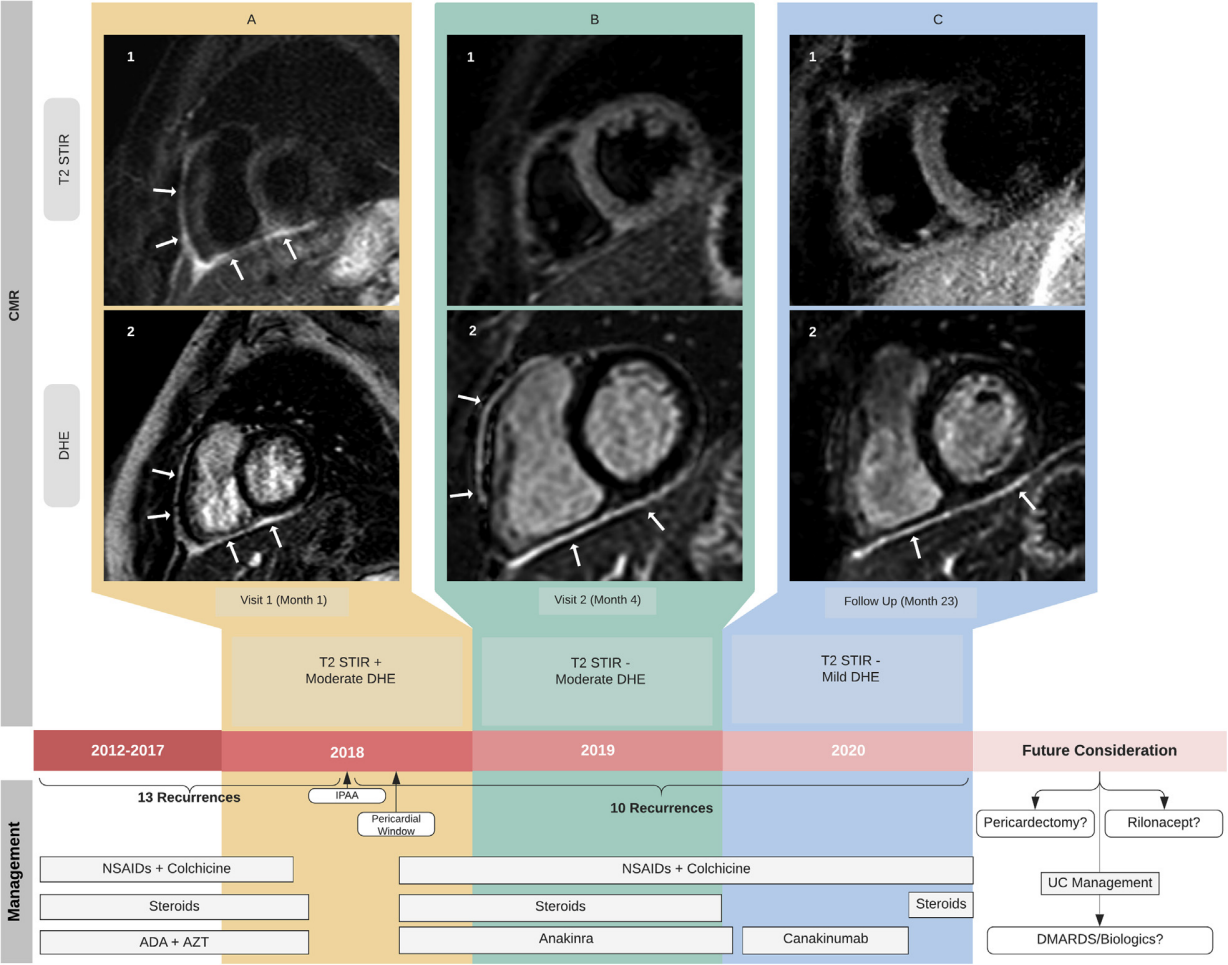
Timeline of Disease With Corresponding CMR Images (A1) Initial CMR demonstrating evidence of increased pericardial signal intensity (arrows) along the anterior and diaphragmatic aspects on T2 STIR imaging, suggestive of active pericardial edema/inflammation. (A2) Pericardial hyperintensity in the same areas on late gadolinium sequences (arrows), overall consistent with acute/sub-acute pericarditis. (B1) No evidence of increased pericardial signal intensity on T2 STIR imaging to suggest pericardial edema. (B2) Persistent moderate pericardial hyperenhancement on late gadolinium sequences (arrows), indicative of sub-acute/chronic pericarditis. (C1) No significant increased pericardial signal intensity on T2 STIR imaging to suggest pericardial edema. (C2) Mild pericardial hyperenhancement on late gadolinium sequences (arrows)*,* predominantly at the diaphragmatic aspect suggestive of ongoing, chronic pericarditis. ADA = adaliumumab; AZT = azathioprine; CMR = cardiac magnetic resonance imaging; DHE = delayed hyperenhancement; DMARD = disease-modifying anti-rheumatic drugs; IPAA = ileal pouch–anal anastomosis; NSAID = non-steroidal anti-inflammatory drug; STIR = short-tau inversion recovery; UC = ulcerative colitis.
